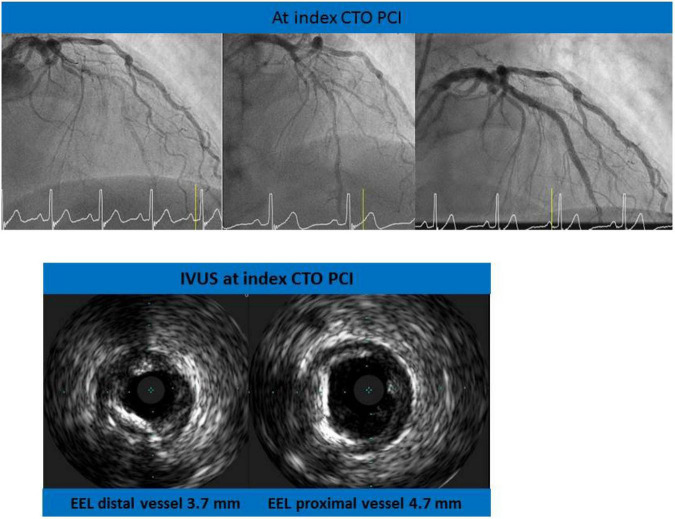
Revascularization of the left anterior descending artery (LAD). Examples of intravascular ultrasound (IVUS) assessment of the chronic total occlusion (CTO) vessel: distal and proximal vessel diameters.
